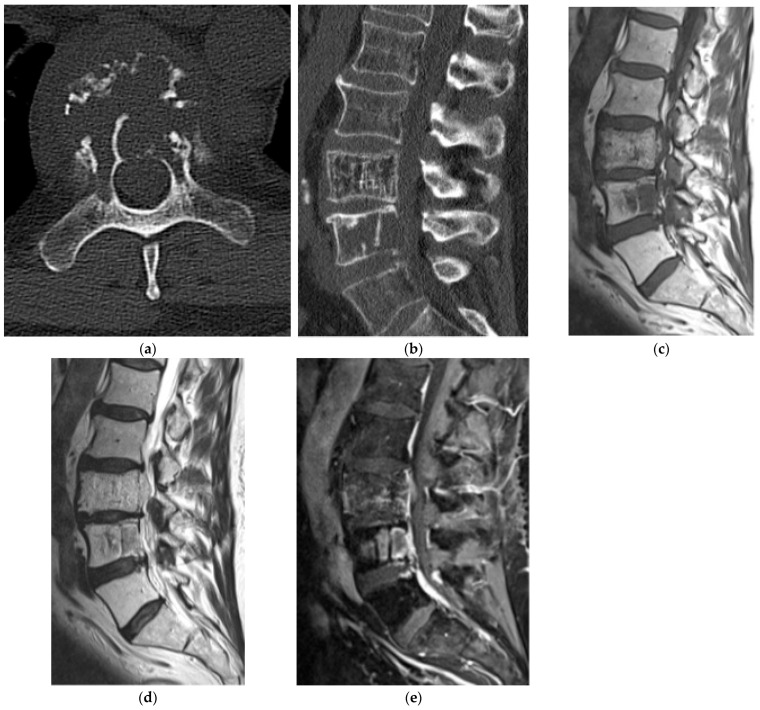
Plasmacytoma. (a) Axial CT bone window, thickened trabeculae with cortical destruction in the L5 vertebral body; this is the characteristic “mini brain” (cerebriform) appearance. (b) Sagittal CT bone window, L4 plasmacytoma with thickened residual trabeculae. Notice a hemangioma in the L3 vertebral body which has thin trabeculae (corduroy sign). (c,d) Sagittal T1 and T2 MR, L4 plasmacytoma with low T1 and T2 signal. (e) Sagittal T1 fat saturation post-contrast, relatively homogeneous enhancement in the plasmacytoma with ventral epidural enhancement possibly representing a combination of epidural disease or venous congestion.
